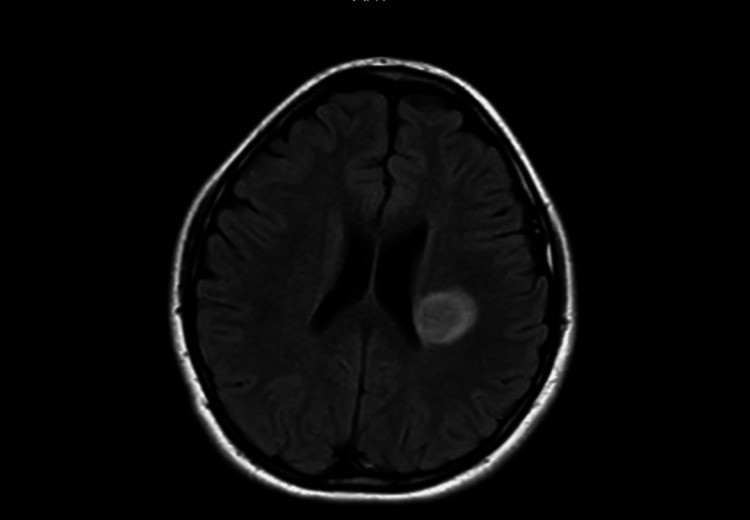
Head magnetic resonance imaging (MRI) shows a single, well-defined T2-flair hyperintense lesion noted in the left centrum semi-ovale, adjacent to the left lateral ventricle. Faintly enhanced compared to the pre-steroid MRI.
